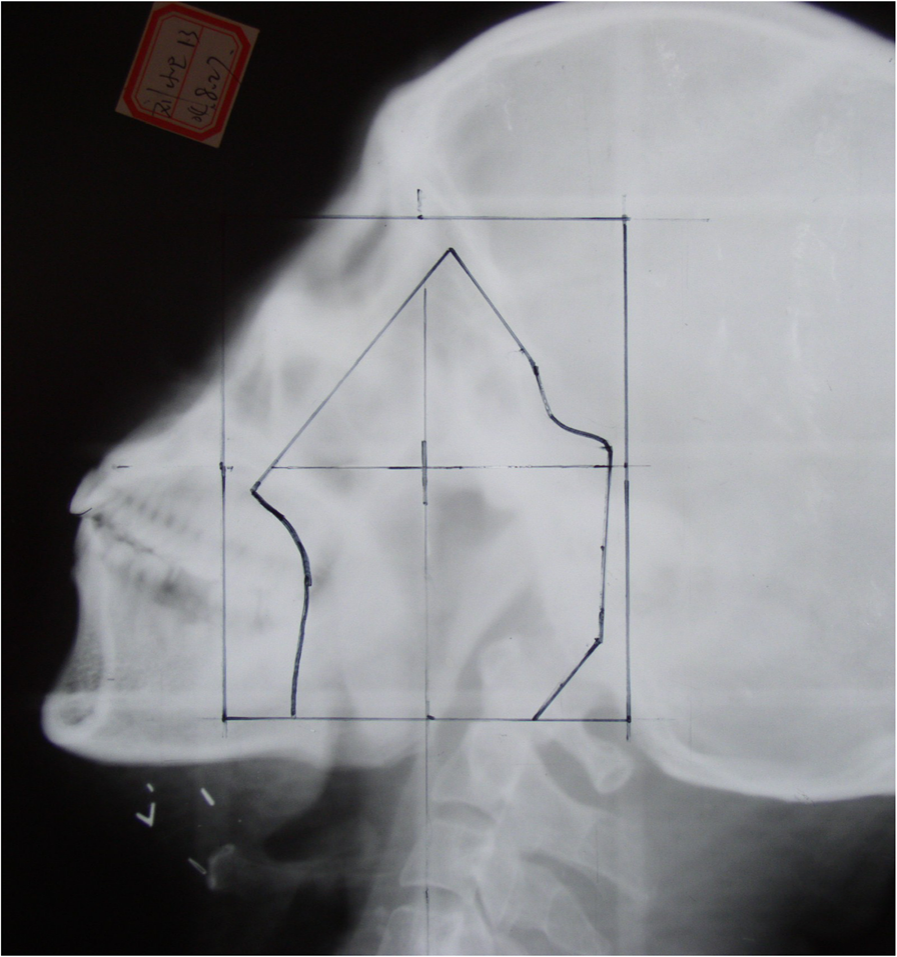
X-ray showing the delineation of the auriculotemporal field in the facial and cervical regions after 19 fractions of radiotherapy (38 Gy); the transferred submandibular gland is outside of the irradiation field.
